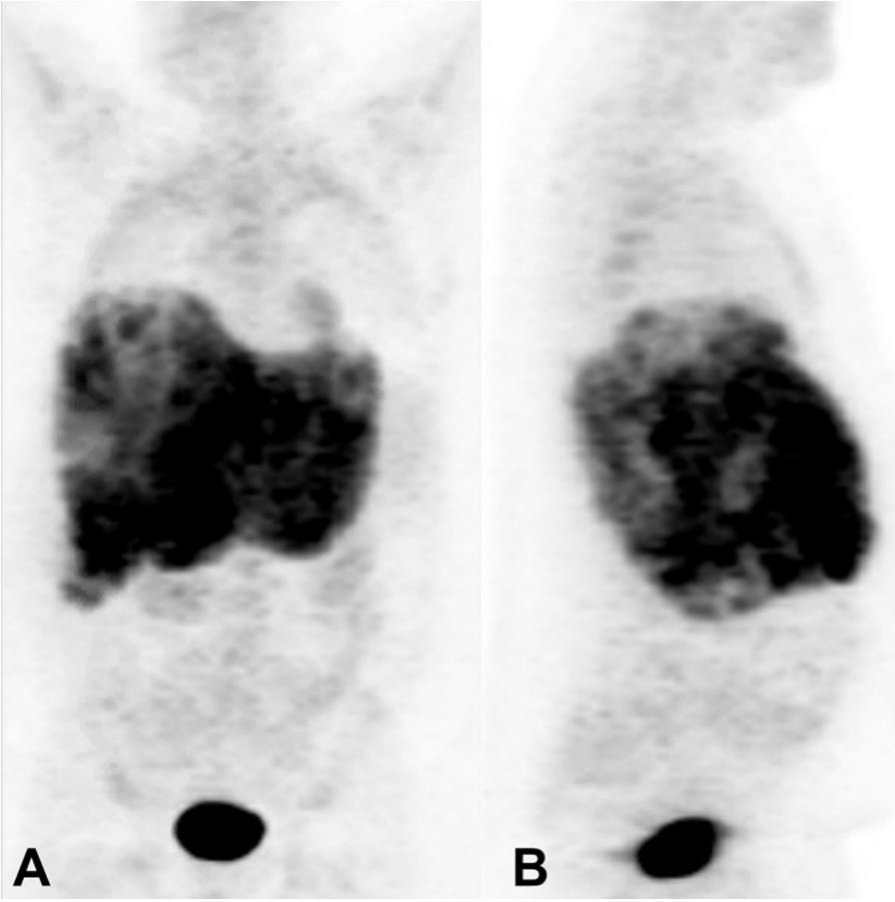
Skull base to mid-thigh PET-CT following IV injection of 18-fluoro-2-deoxyglucose (FDG) with standard uptake period A ­ coronal and B ­ sagittal views. Hypermetabolic thickening involving the lesser curvature of the stomach is observed, as well as numerous hypermetabolic hypodensities are throughout the liver and lymph nodes.
